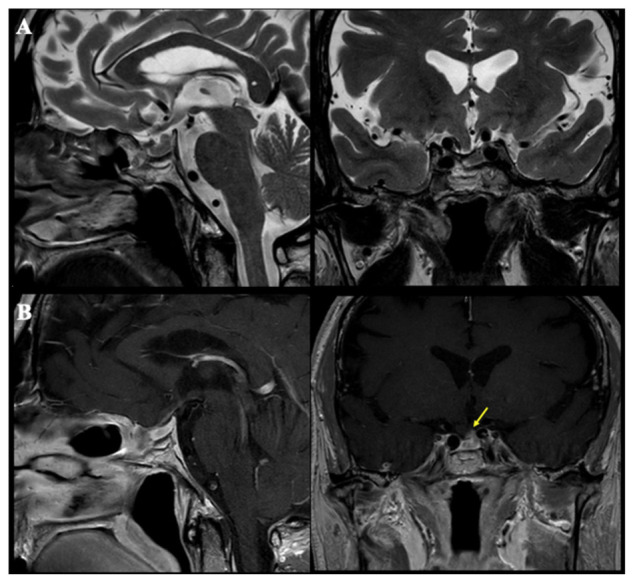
One-year postoperative magnetic resonance imaging. (A) Sagittal (left) and coronal (right) T2-weighted images demonstrate the postoperative appearance of the sellar region without evidence of recurrent mass. (B) Contrast-enhanced sagittal (left) and coronal (right) T1-weighted images demonstrate a stable 3 × 2 mm nodular enhancing focus at the operative site (yellow arrow), without interval progression or radiological evidence of tumor recurrence.
